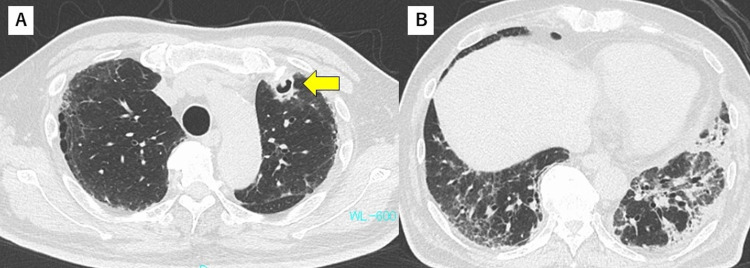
Chest CT findings on day 42 (A) Gradual improvement of the cavity was observed (arrow). (B) Lung fibrosis improved with partial lung shrinkage.
